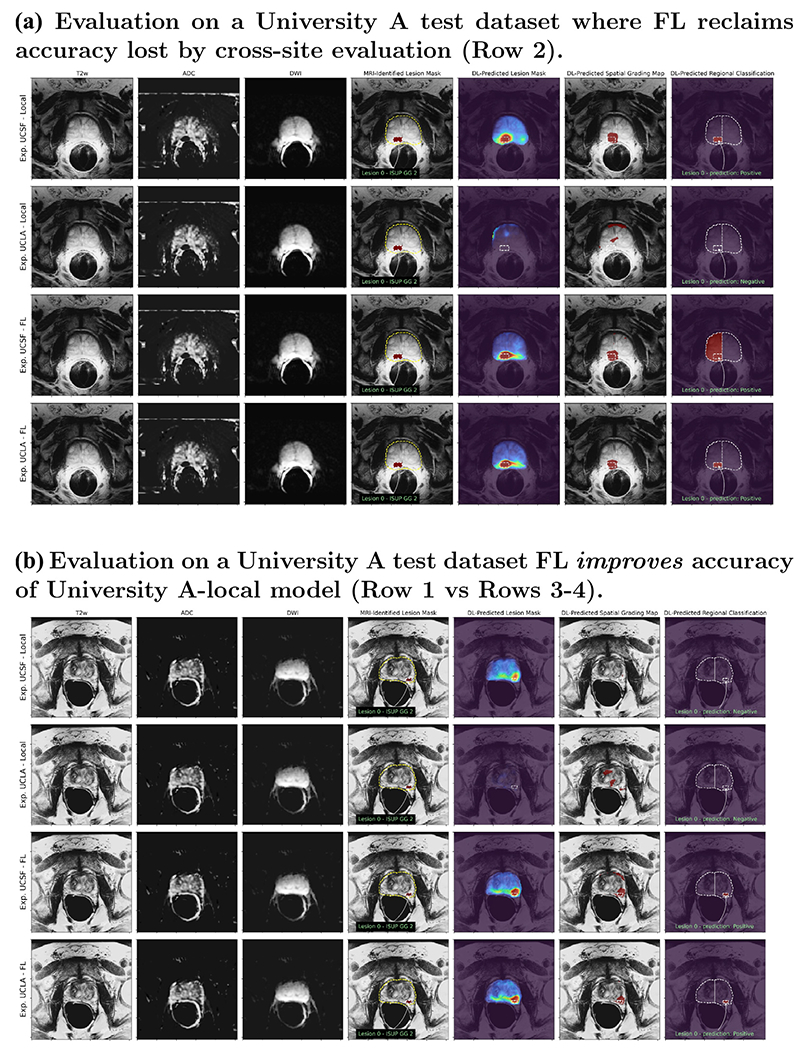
Evaluation of UCNet models on example UCSF dataset. (A) depicts a transverse slice of an exam with a MRI-identified ISUP GG 2 lesion where the UCLA-local model performs poorly, but both federated models (Rows 3-4) achieves the same level of accuracy as UCSF-local model. (B) depicts a transverse slice of an exam with a MRI-identified ISUP GG 2 lesion where both local models perform poorly, but federated models correctly classify this lesion as CS-PCa. Notably, for both (A-B), federated checkpoint chosen by UCLA stopping criteria (Row 3) performs better than the checkpoint chosen by UCSF (Row 4), highlighting that neither site has sufficient data to generalize well on their own. (Color version of figure is available online.)
